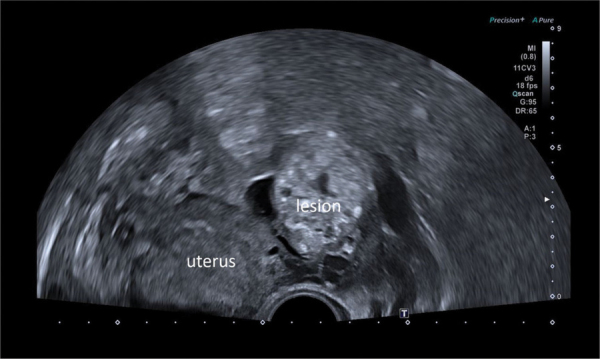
Ultrasound showing nodular lesion with hyperechoic spots and cyst-like areas. Lateral to the uterus.
